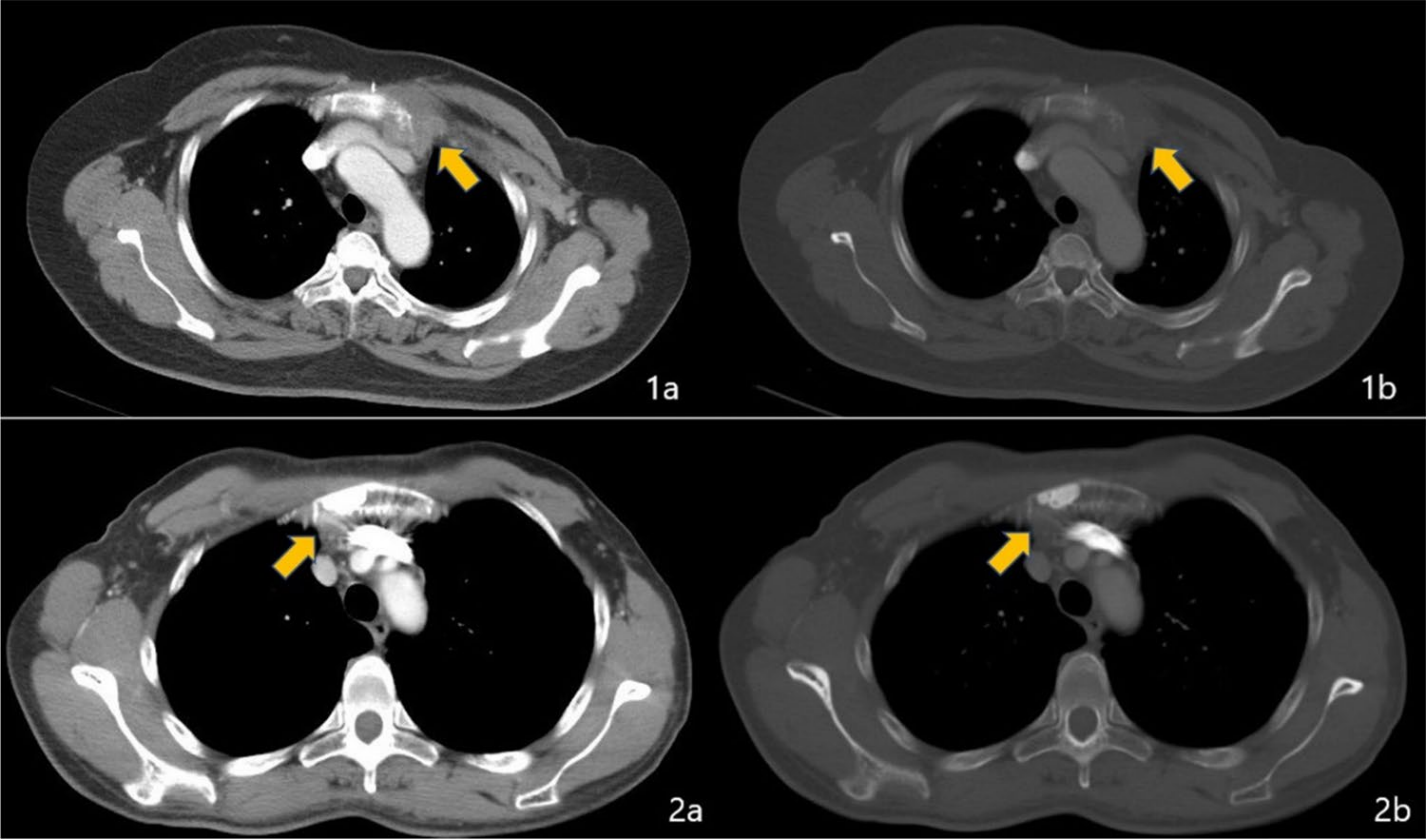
Computed tomography reveals internal mammary lymph node metastasis (yellow arrow) with sternal erosion, sternal metastasis (yellow arrow), and a local soft tissue lump. Views are shown in the mediastinal window (a) and bone window (b)
